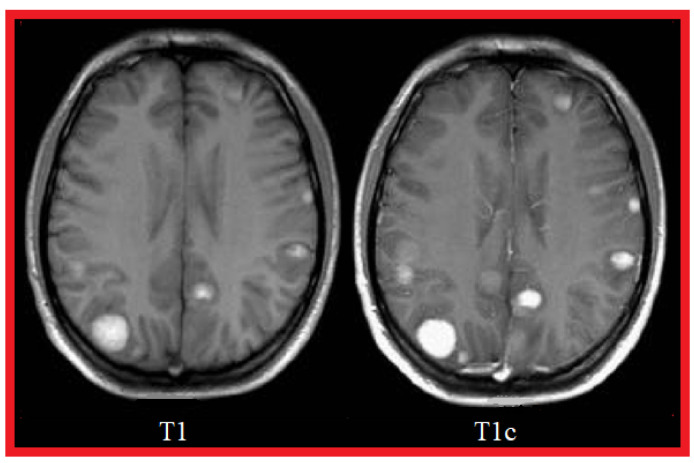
Comparison between a T1-Weighted MRI sequence without a contrast agent (T1) and the same sequence with a contrast agent (T1c).
